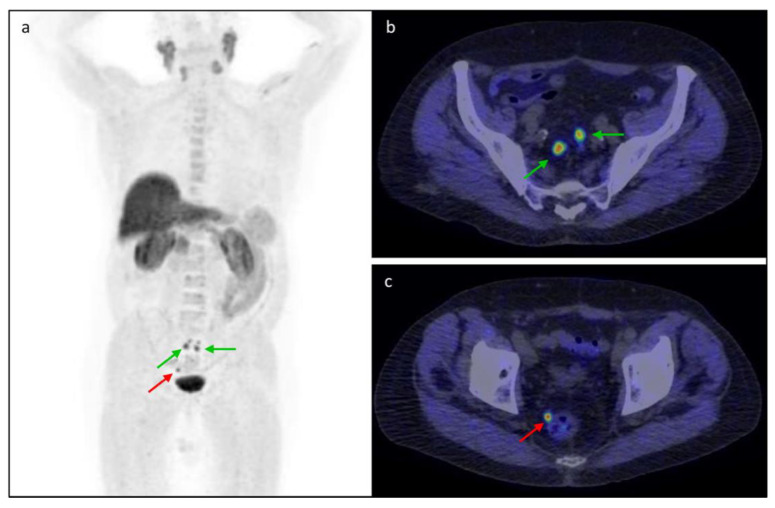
An 84-year-old patient with PSA increase (2.97 ng/mL) and low PSAdt (3.5 months) after prostatectomy (Gleason Score 8 = 4 + 4) was evaluated with the [^18^F]fluorocholine PET/CT. The (a) MIP and (b,c) axial fused images showed recurrent lymph node lesions, namely (b) two internal iliac nodes with SUVmax 8.9 (green arrow) and (c) one right perirectal node with SUVmax 10.3 (red arrow).
